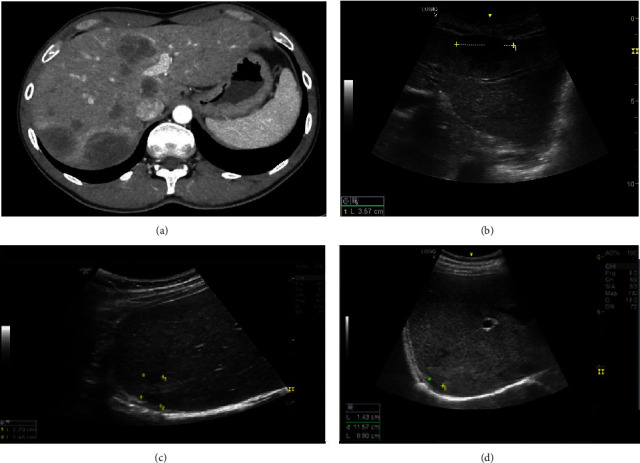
(a) A contrast-enhanced CT scan of the patient at the time of diagnosis. (b) A hypoechoic, round, well-defined lesion at the time of the first US scan. (c, d) US scan after treatment showing involution of the hypoechoic lesions shown at the first US.
